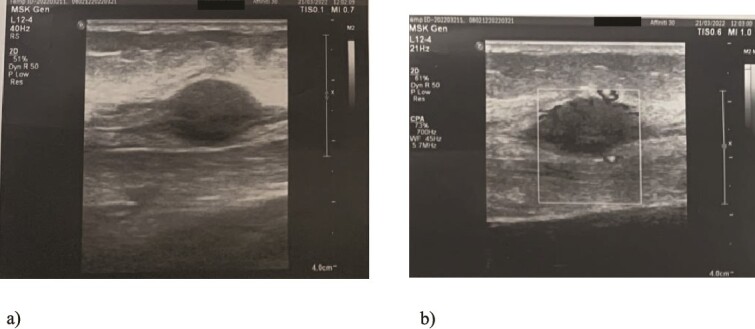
Ultrasound examination of the tumor: Spindle-shaped hypoechogenic and subcutaneously placed mass (a) with marked hypervascularity on color flow mapping (b).
